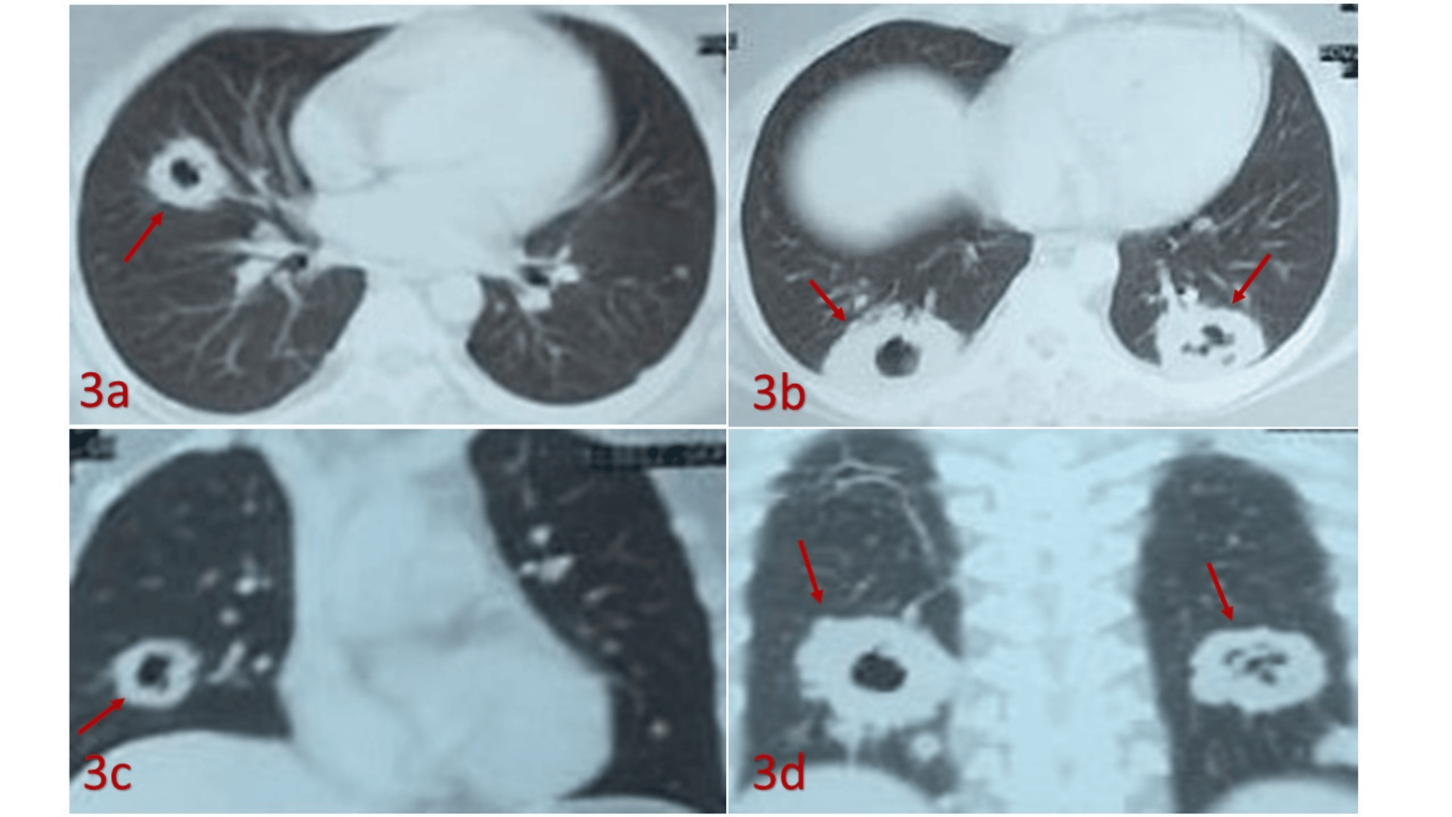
High-resolution computed tomography (HRCT) chest images (a–d) showing characteristic pulmonary findings in GPA Axial (3a, 3b) and coronal (3c, 3d) views reveal multiple thick-walled cavitary nodules (red arrows) in both lungs. These findings are suggestive of necrotizing granulomatous inflammation, typical of GPA-related pulmonary involvement. GPA: granulomatosis with polyangiitis
